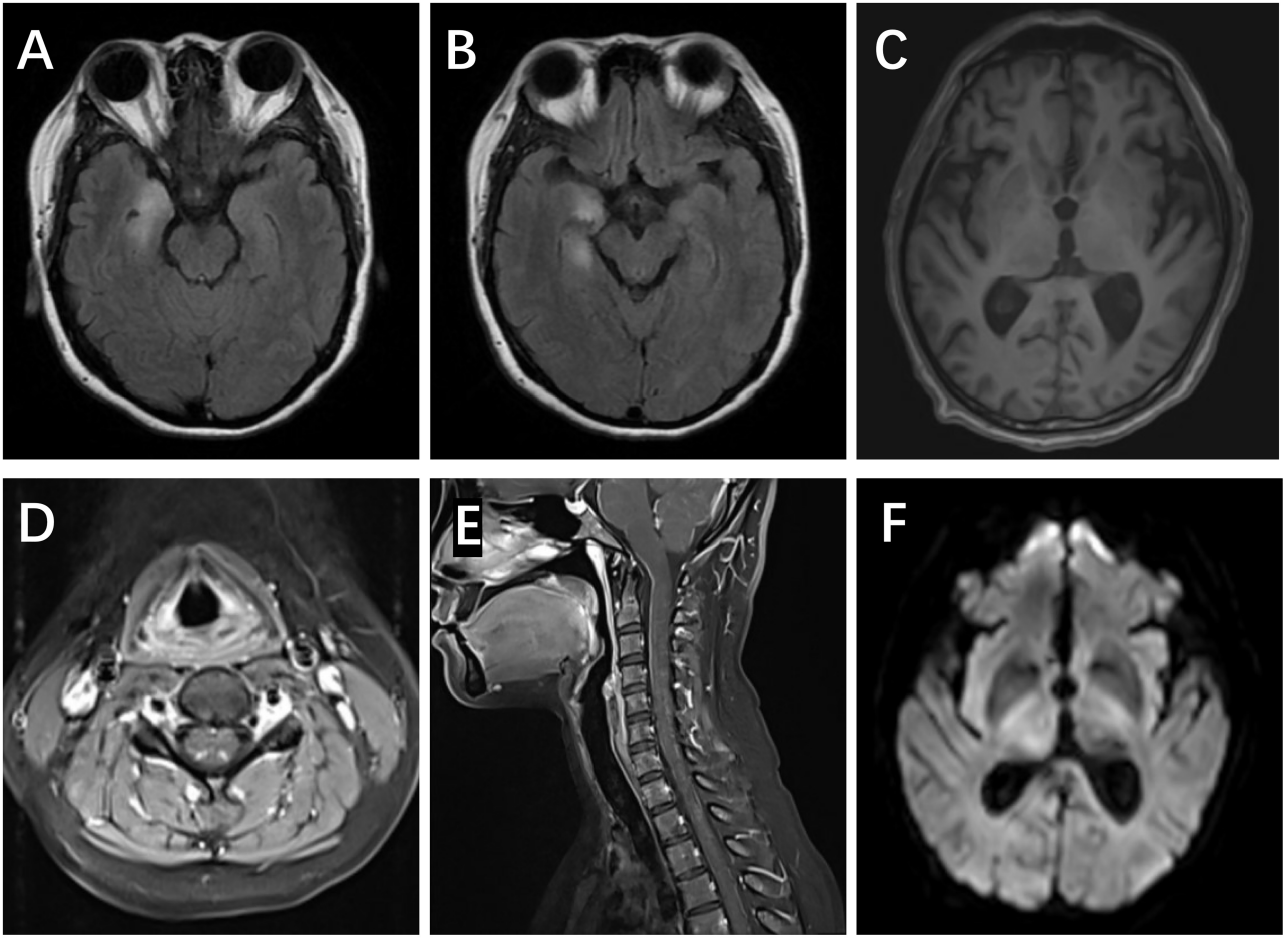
MRI scans in anti-amphiphysin encephalitis. (A, B) Right FLAIR hyperintensities in the medial temporal lobes, including both the amygdala and hippocampus (Patient #1). (C) The blurred structure of the bilateral thalamus (Patient #4). (D, E) Increased T2 signal and enhancement in posterior horn of the spinal cord (C2-7) (Patient #9). (F) Restriction of DWI in the right thalamus (Patient #4).
